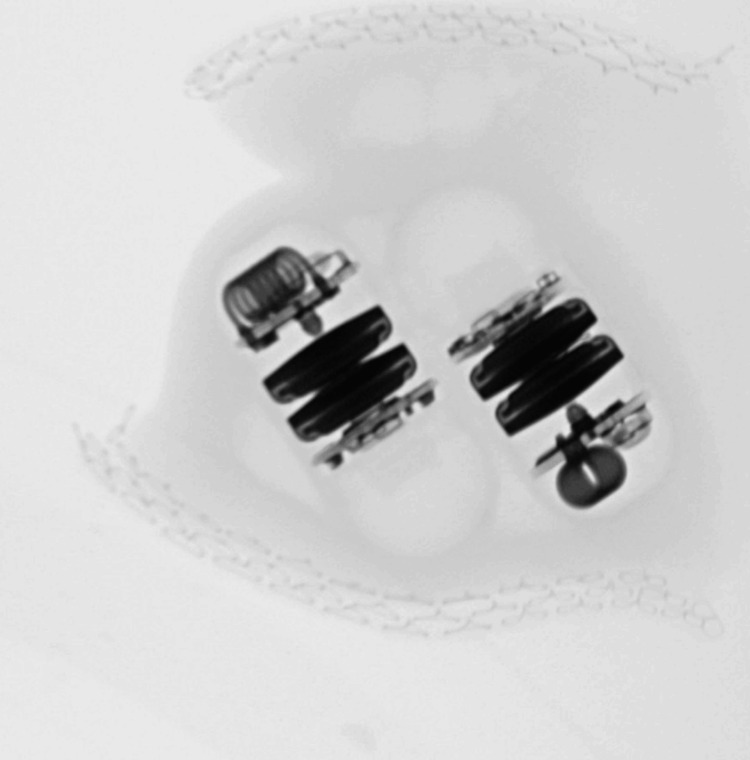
Intraoperative X-ray of video capsules post-resection.
